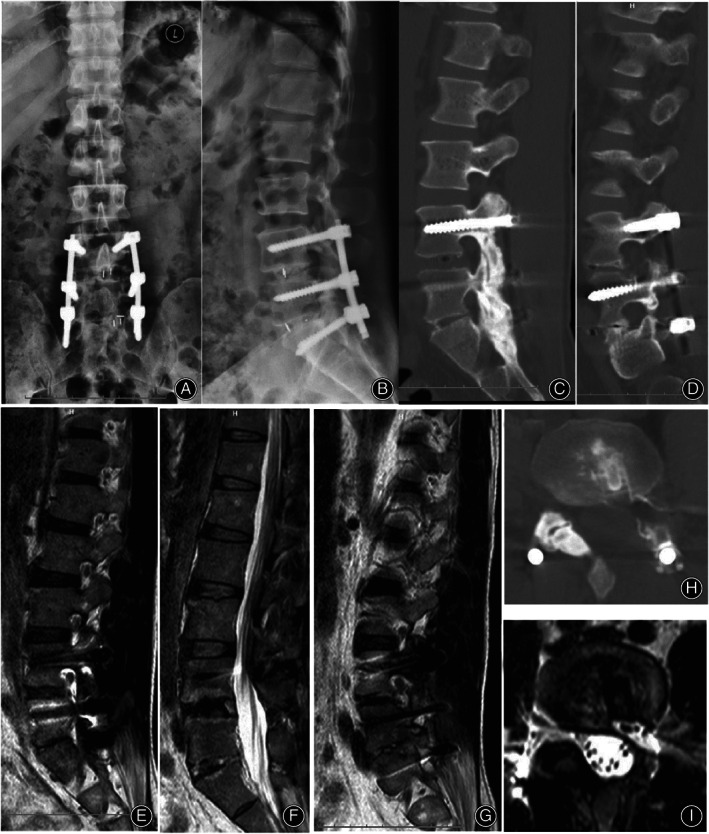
Imaging material of post‐operation this time. (A, B) Postoperative X‐ray review; (C, D, H) Postoperative CT scan review; (E–G, I) Postoperative MRI review
